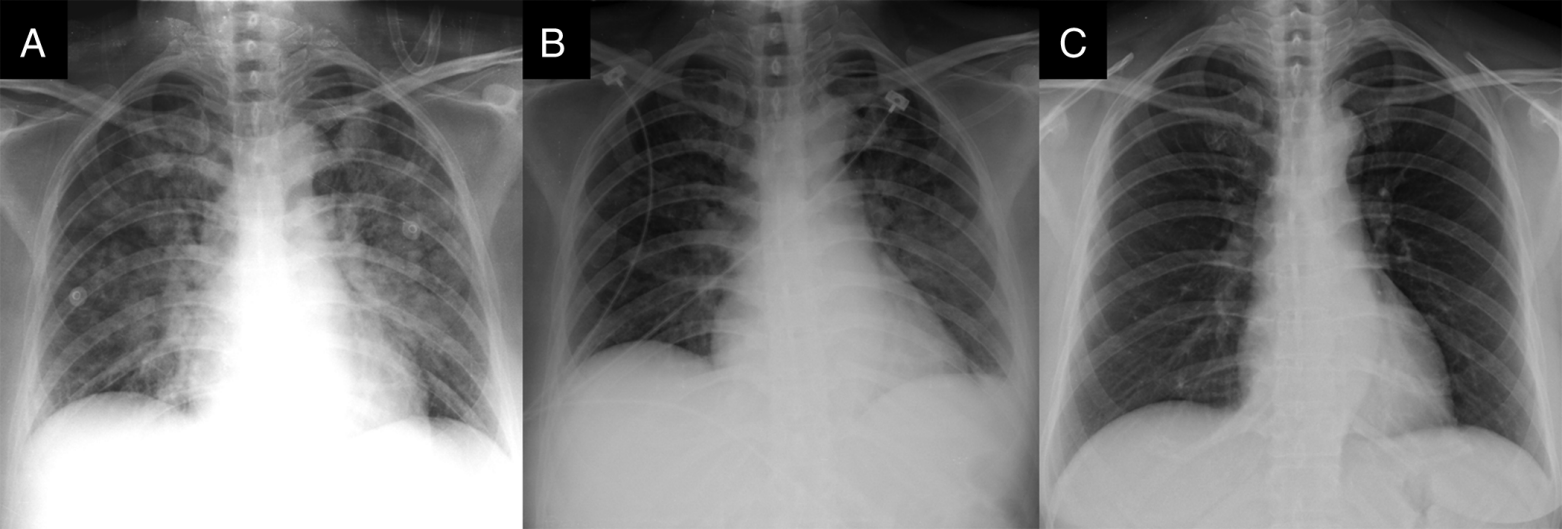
The serial chest radiographs: (A) breathlessness onset on day 1; (B) under bilevel positive airway pressure ventilation on day 3; (C) discharge on day 6.
